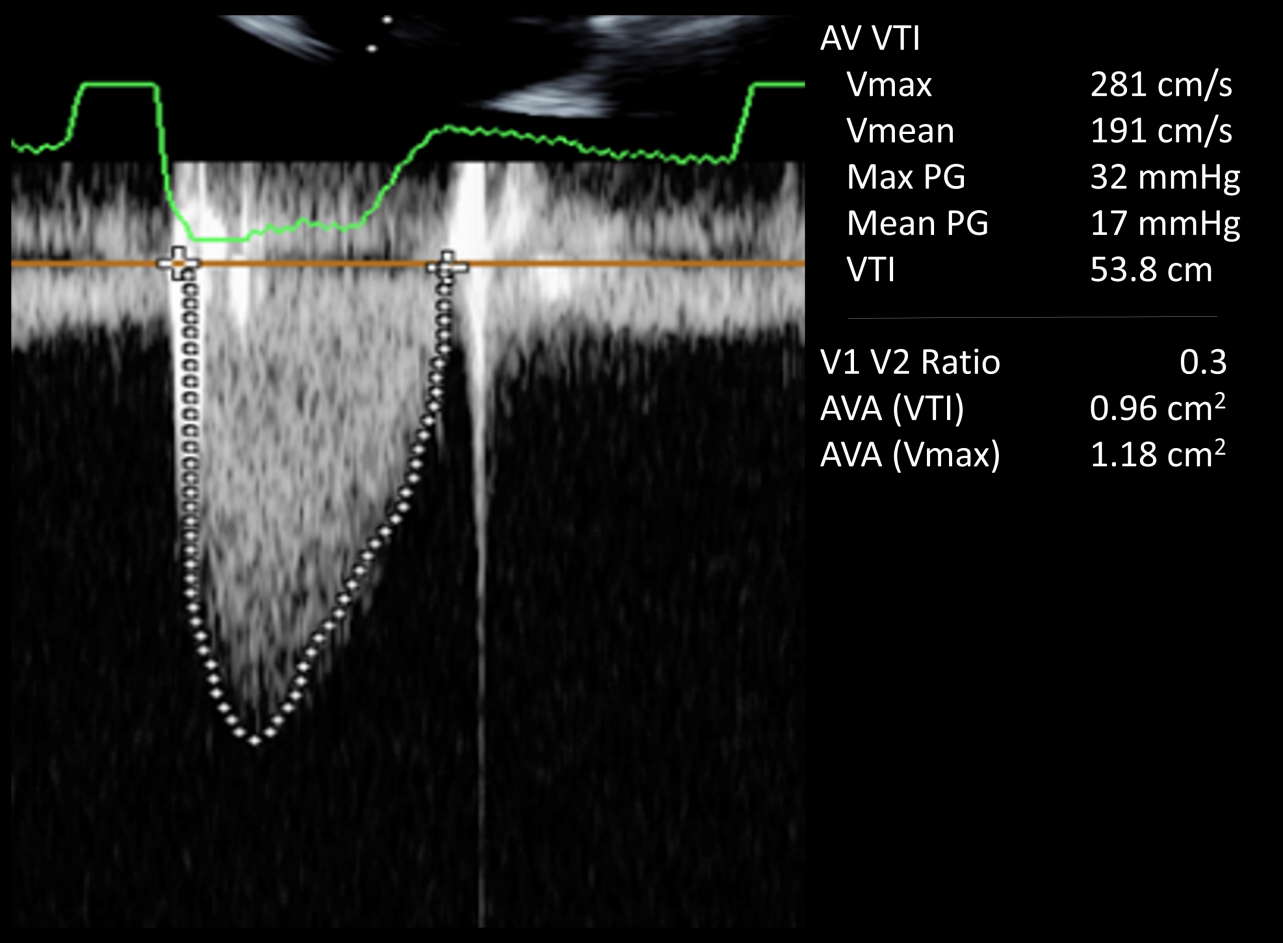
Doppler echocardiogram showing improvement in peak and mean gradients at 32 mm Hg and 17 mm Hg respectively with treatment of anemia
